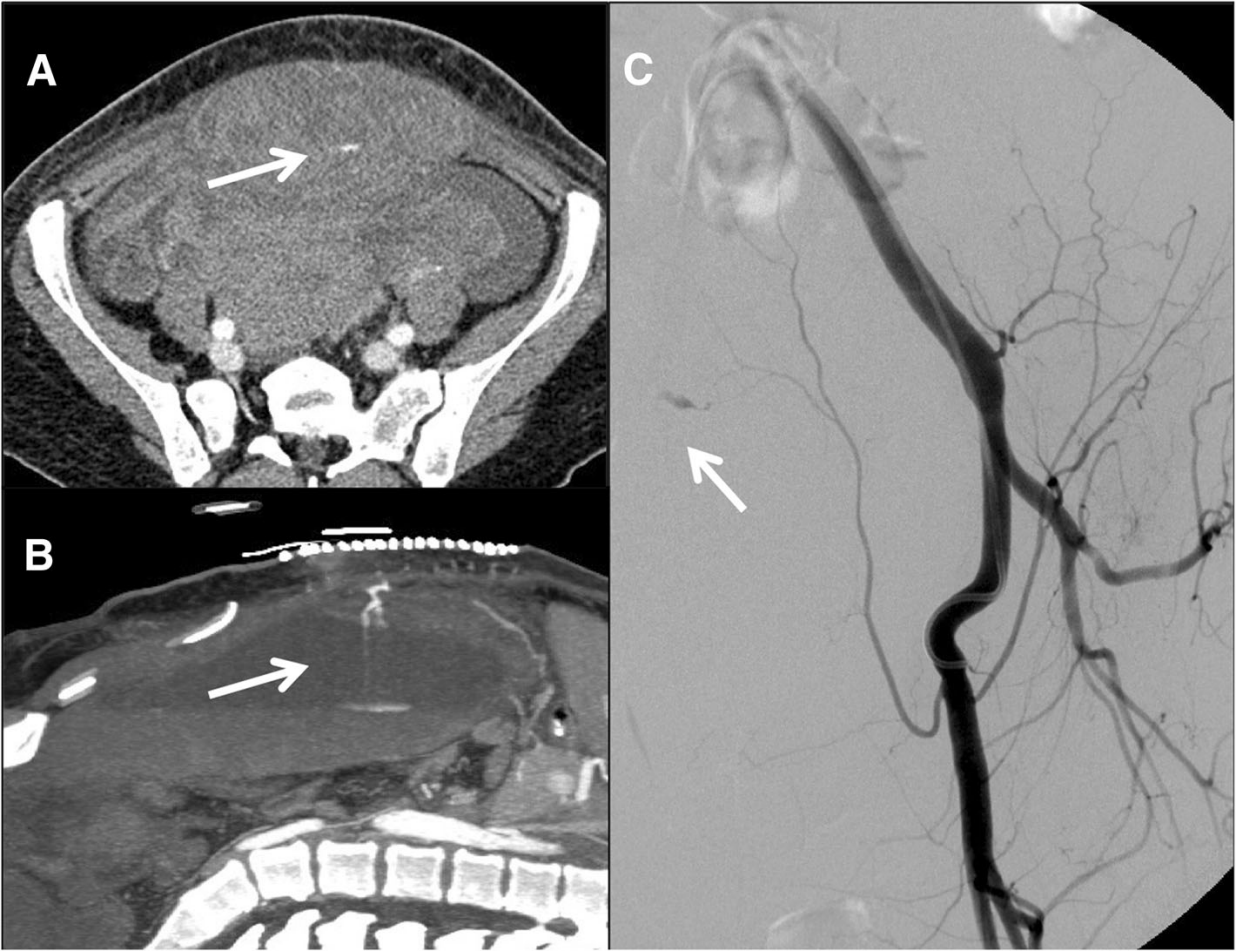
(Same patient of Fig. 3). Contrast-enhanced CT (a, b) showed contrast material extravasation (white arrow) suggestive for active bleeding confirmed by arteriography (c)
